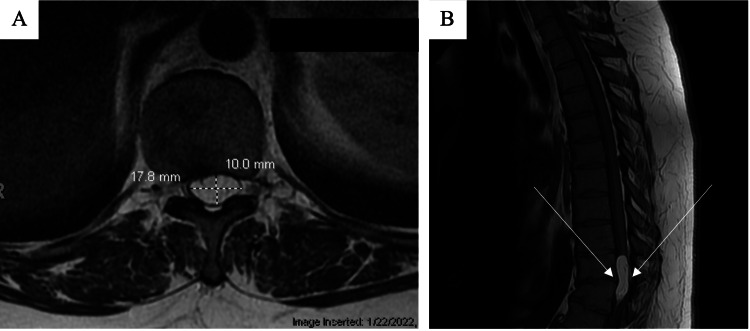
Pre-operative MRI of the spine A) T1 sagittal view MRI of L-spine showed an intradural 1.1 x 1.4 x 4.0 cm mass at the distal thoracic cord level at T10 and T11. B) T2 axial view showed hyperintense lesion consistent with a diagnosis of thoracic lipoma.
